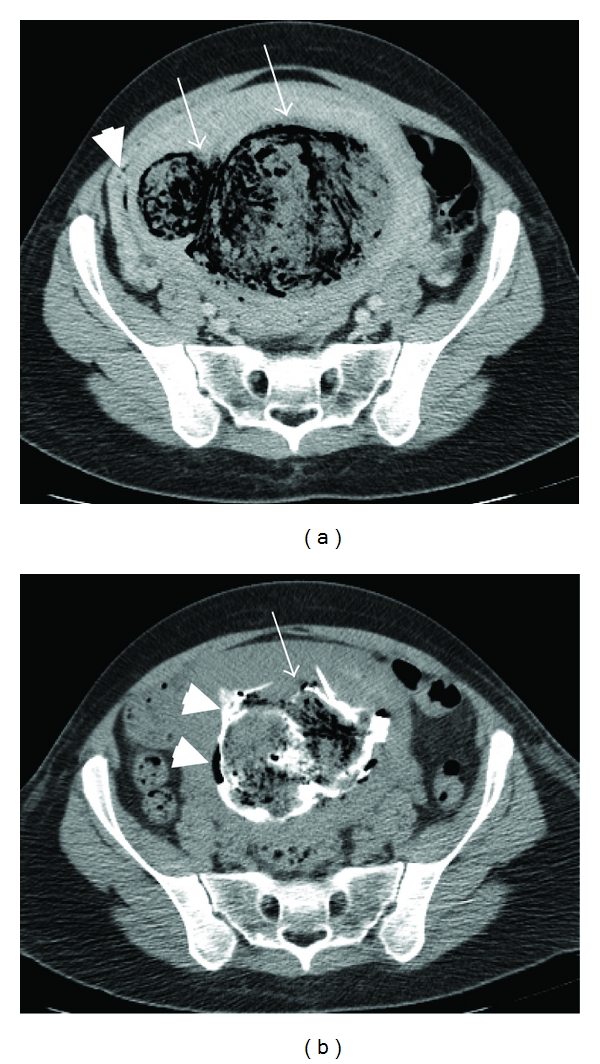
CT of the pelvis demonstrating (a) a large lobular gas containing intramural mass in the left uterine wall (white arrows). The uterine cavity is compressed to the right side (white arrowhead). (b) Following the injection of contrast material into the mass, the contrast is filling the mass (white arrow) and the uterine cavity (white arrowheads).
